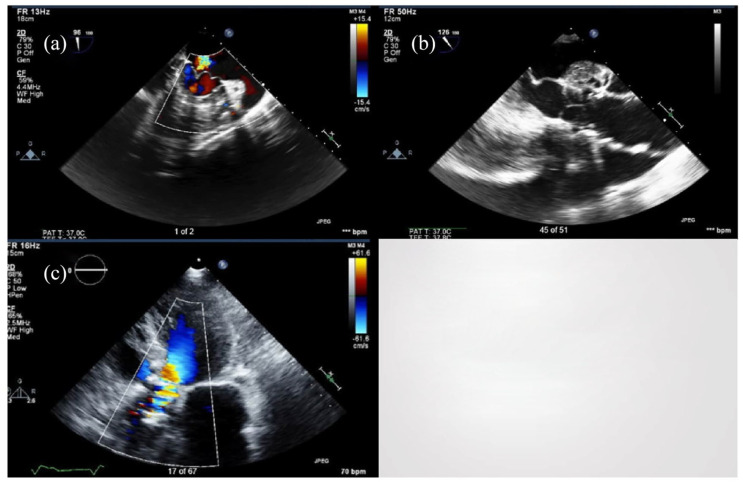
Preoperative echocardiogram of the aortic valve (a and b) and a postoperative
